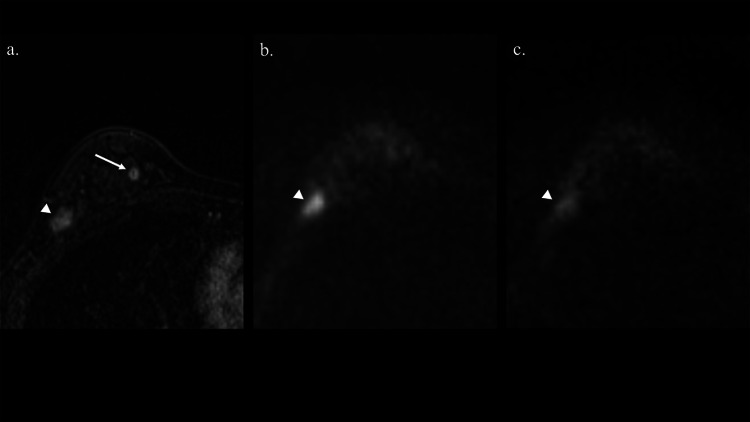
A 53-year-old woman underwent breast MRI for evaluation of a lesion detected on mammography and ultrasonography in the right breast. The index lesion (a–c; arrowheads) was pathologically diagnosed as fibroadenoma. On early-phase DCE-MRI, a 5.2-mm MRI-detected mass was identified in the ipsilateral breast (a, arrow) and assessed as BI-RADS category 4. On both DWI (b, DWI_800_ and c, DWI_1500_), the lesion was isointense to the surrounding fibroglandular tissue and not visually distinguishable (b, c, arrow). Both readers assigned a DWI visibility score of 0 on both DWI. On MRI-targeted ultrasonography, the lesion was depicted as a 6-mm mass and was diagnosed as fibrosis by ultrasound-guided core needle biopsy (DWI true-negative)
